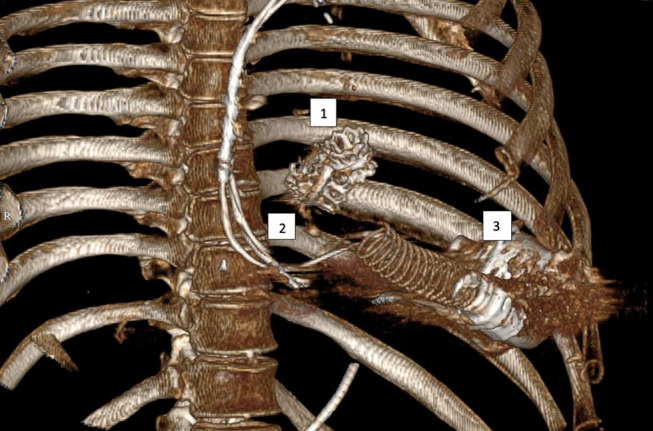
Chest CT after the procedure. 1. AV projection: valve in frame – MyVal (Meril) 21.5. 2. MV projection: valve in frame – MyVal (Meril) 27.5. 3. LVAD–Heart Mate 3.
